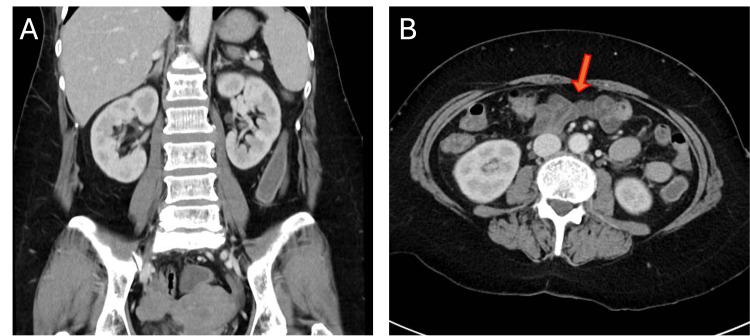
CT of the abdomen with contrast at her first presentation A: On the sagittal plane of the contrast-enhanced computed tomography (CECT), there is no evident contrast enhancement surrounding the psoas muscles or perinephric area. Although a compression fracture at L5 is observed, there are no surrounding contrast-enhancing lesions. B: In the axial plane of the contrast-enhanced CT, fluid retention is noted within the small intestine, and no other significant findings were observed.
